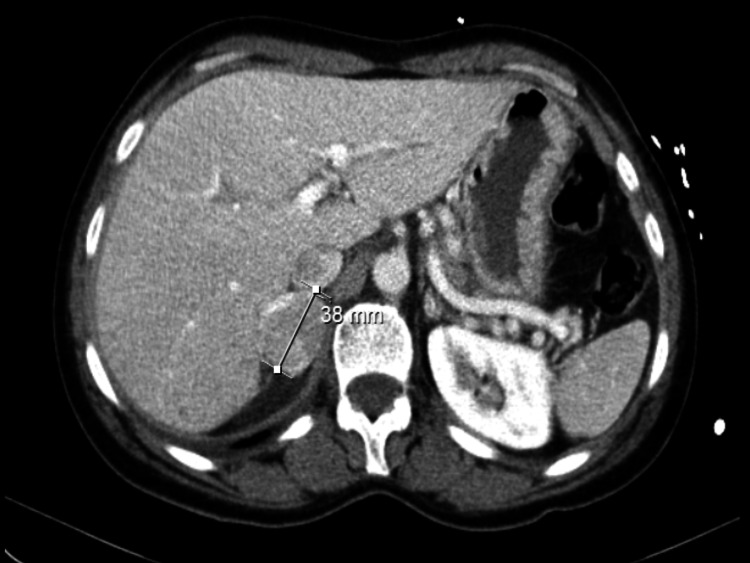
Abdominal CT scan revealing a right adrenal nodule suggestive of pheochromocytoma This CT scan shows the right adrenal nodule, which played a crucial role in diagnosing the patient's pheochromocytoma CT, computed tomography
